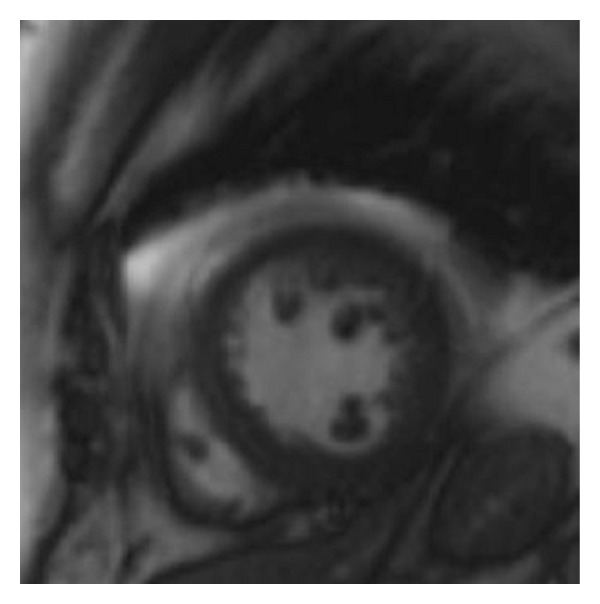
Mid cavitary view of the left and right ventricle on cardiac MRI.
